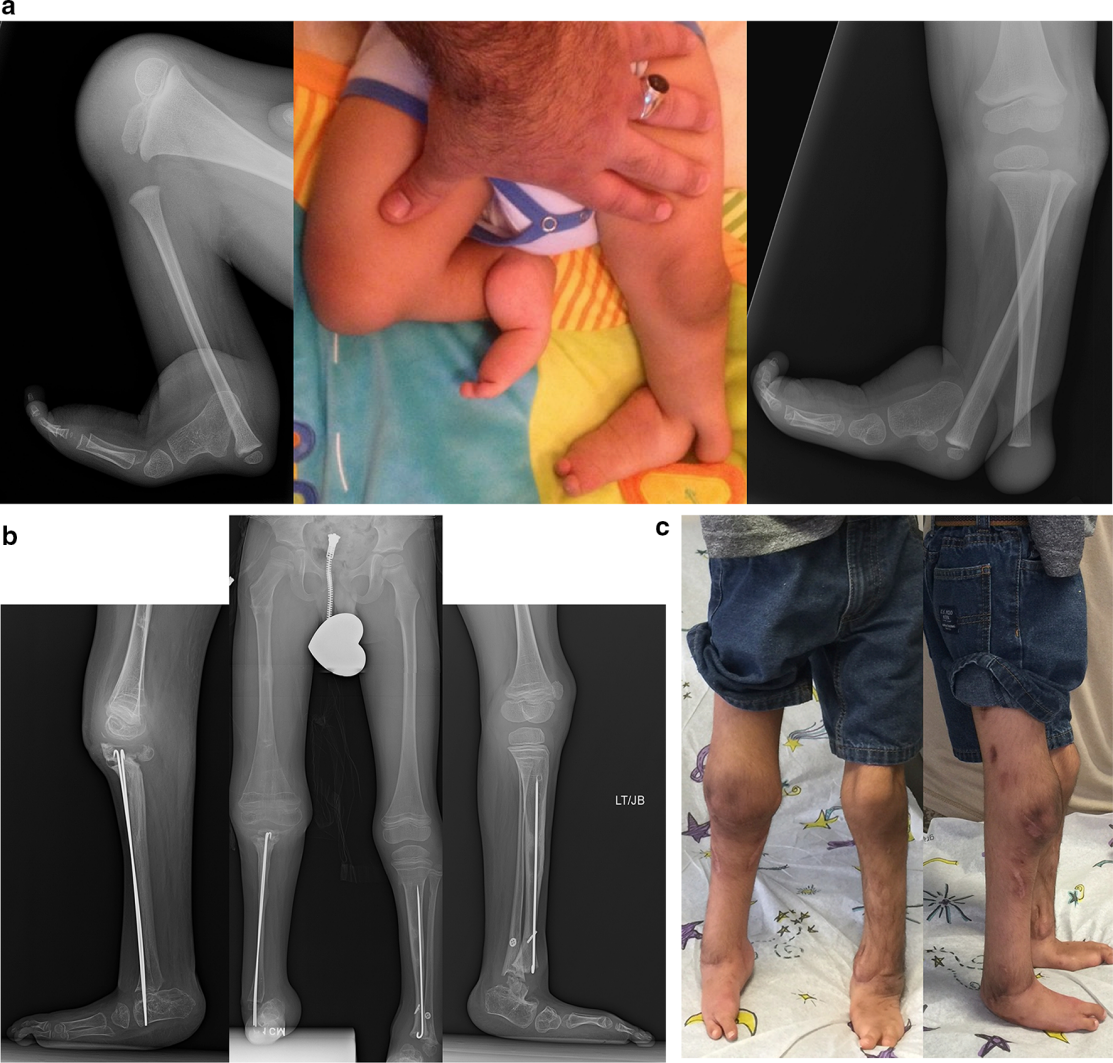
a Preoperative photograph (*center*), and radiographs (*right* on the *left*, *left* on the *right*) of bilateral tibial hemimelia in a 3-year-old boy; Paley type 5A (*right*) and Paley type 3B tibial hemimelia (*left*). The patella on the *right* has not yet ossified. Other than the cleft on the *left*, this is very similar to type 3A. The cleft makes this a very rare type. It should be noted that the foot is found on the fibular side of the cleft on the *left*. Both feet are in severe equino-varus and even upside down. There is a greater than 90° flexion contracture and dislocation at the right knee. b Standing (*center*), right lateral (*left*) and left lateral (*right*) radiographs after the staged reconstruction method was completed, 2 years prior on the *left* and 3 months prior on the *right*. The Paley–Weber patellar arthroplasty was performed on the *right*, with insertion of BMP2 to ossify the patella and fuse it to the proximal fibular epiphysis. Note the preservation of the proximal fibular physis. On the *left* the talus was brought under the tibia, a biologic arthroplasty performed together with plastic closure of the cleft. The distal and proximal tibial and fibular physes are preserved on both sides. The fusion of the talus to the distal fibular epiphysis did not occur and may require revision in the future. Both feet are plantigrade. c Clinical photographs showing the patient standing with both feet plantigrade and both knees straight and well aligned
